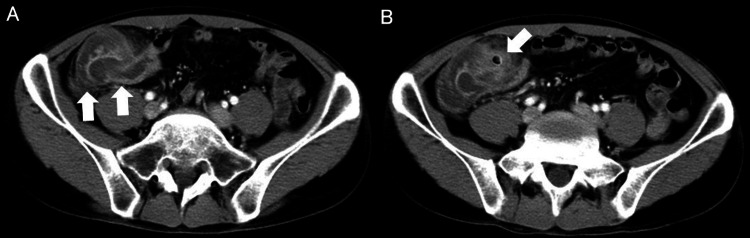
Abdominal CT. (A) Contrast-enhanced CT shows ileocolic intussusception with concentric mural thickening of the terminal ileum and cecum and surrounding inflammatory fat stranding (arrows). (B) An intraluminal structure with a centrally hypoattenuating core and hyperattenuating rim is visible (arrow) CT: computed tomography
